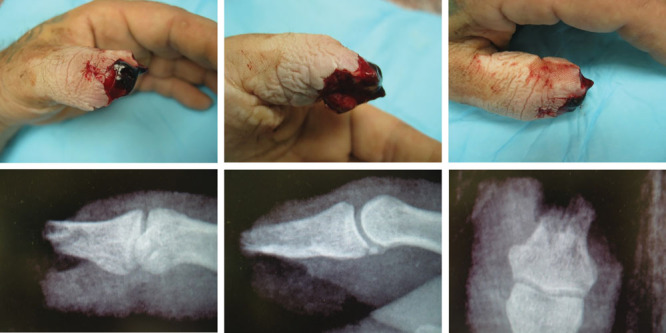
These are 3 views of an amputated thumb tip and its x-rays. One can see that the bone is clearly exposed. However, the fat, skin, blood vessels, and nerves were gradually pulled over the exposed bone over 6 weeks with wound contracture. Every day, the wound was showered and covered with Vaseline and Coban tape so the raw wound did not dry and die.
